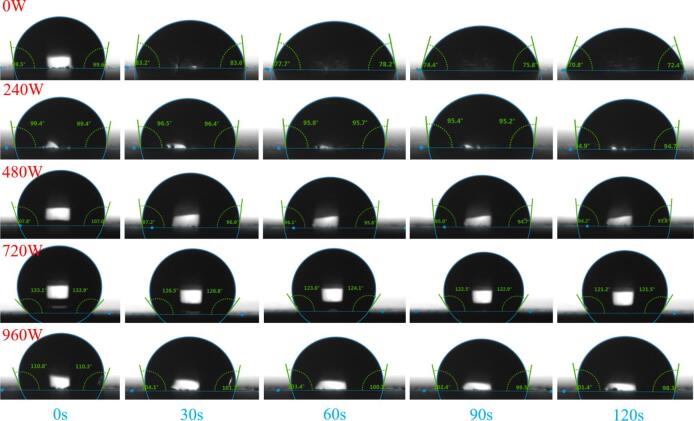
Variation in the contact angle with time for different ultrasonic power groups.
